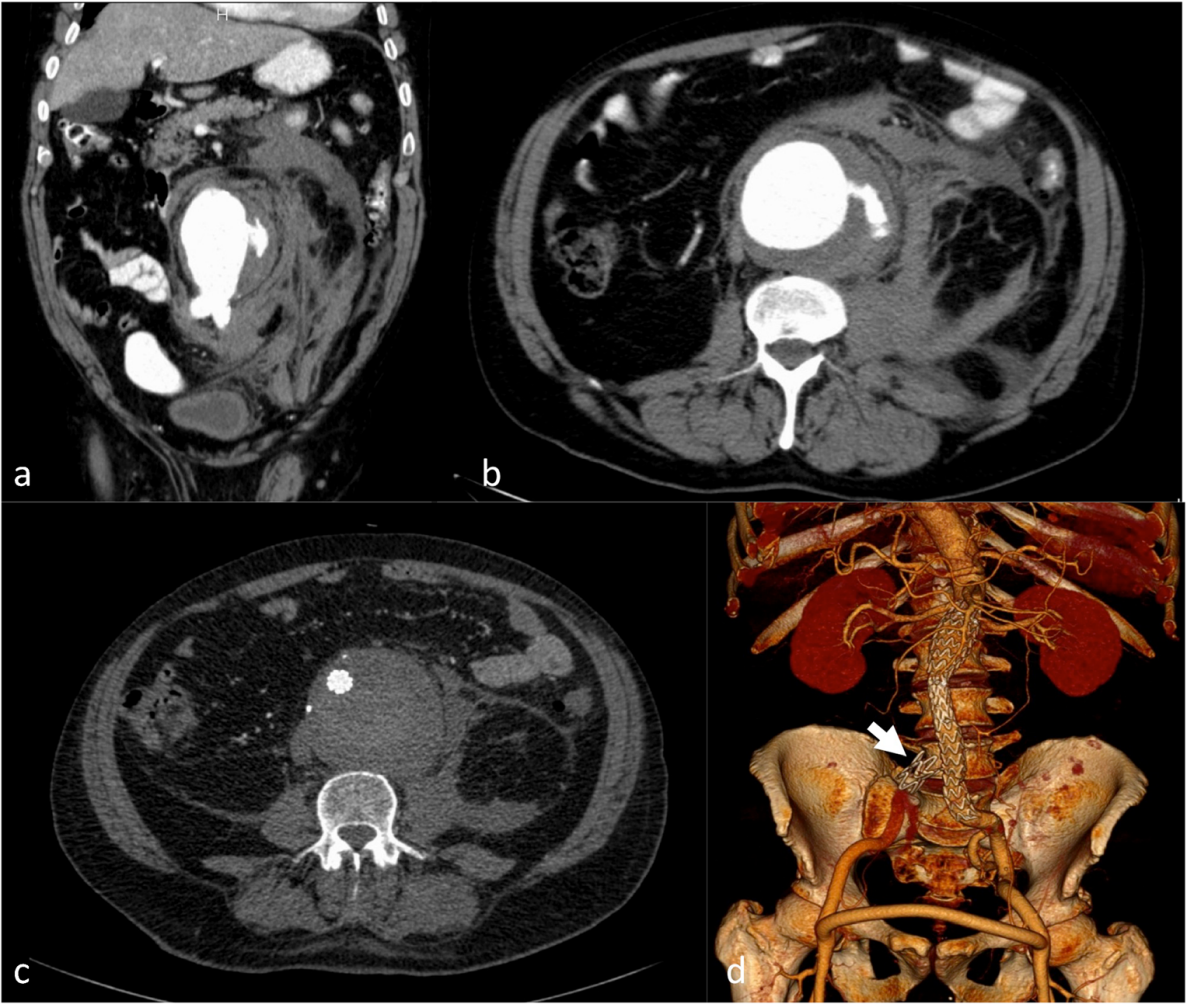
Ruptured abdominal aortic aneurysm with stent graft treatment. The 72-year-old male patient presented with left-sided abdominal pain and hypotension. CTA demonstrated a ruptured infrarenal aortic aneurysm on coronal and axial sections (a–b). The patient was treated with aorto-uni-iliac stent graft implantation (d) to the left, a crossover bypass and placement of an occluder (*arrow*) in the right common iliac artery. Follow-up CTA (c) demonstrated complete exclusion of the large aneurysm
